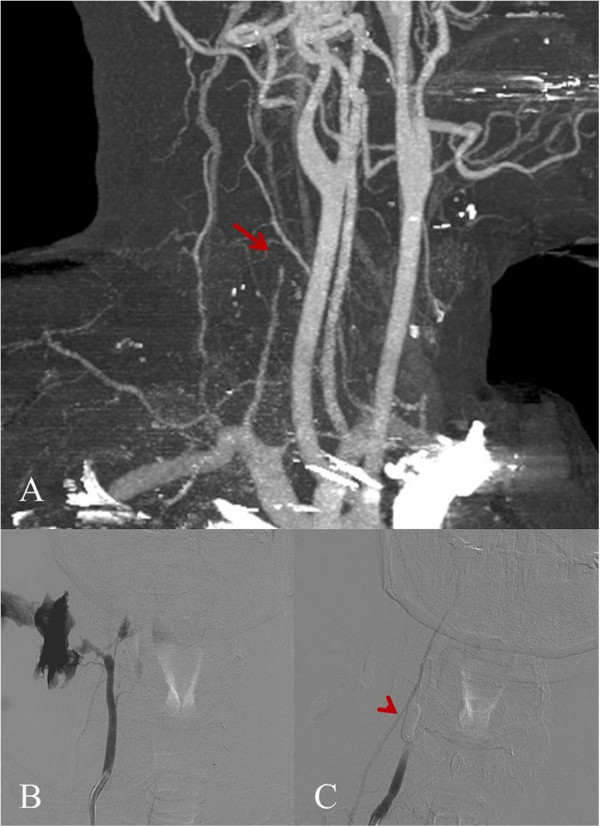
Anterolateral view of CTA (A) and frontal projection of right vertebral artery on conventional angiogram (B and C). A. Tapering of the right vertebral artery (VA) is shows with the arrow. B. The bleeding, which started during navigation to the right VA. C. The stable situation after inflation of the detachable balloon is shown with the arrowhead.
